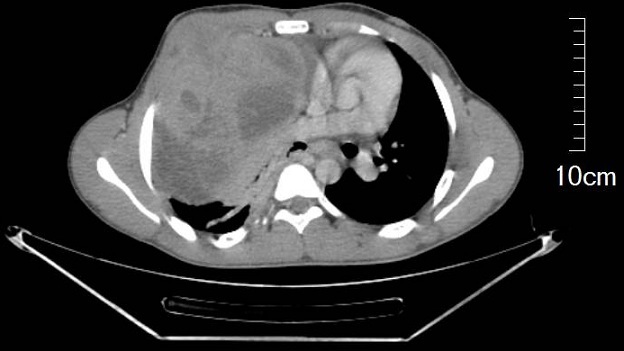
Contrast-enhanced chest CT showing a heterogenous mass in the right lung with infiltration of the soft parts of the chest wall
